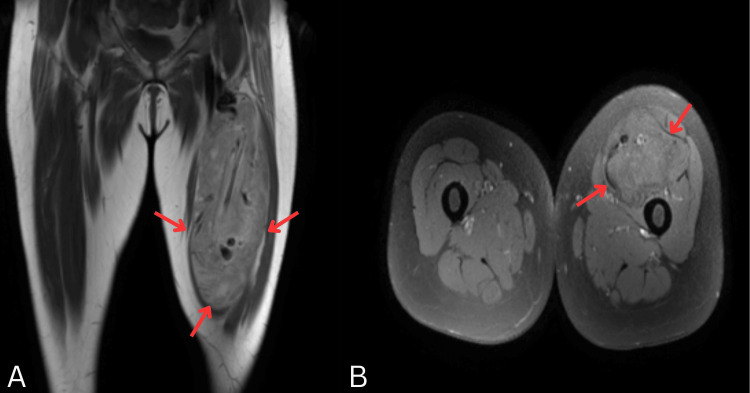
MRI of the left thigh demonstrating an intermuscular mass (A) Coronal T1-weighted image shows a well-defined heterogeneous intermuscular lesion in the anteromedial compartment (arrows), containing areas of fat signal and internal vascular channels. (B) Axial T1-weighted image highlights a large heterogeneous mass with prominent vascular structures in the left thigh (arrows), in contrast to the normal appearance of the right thigh.
